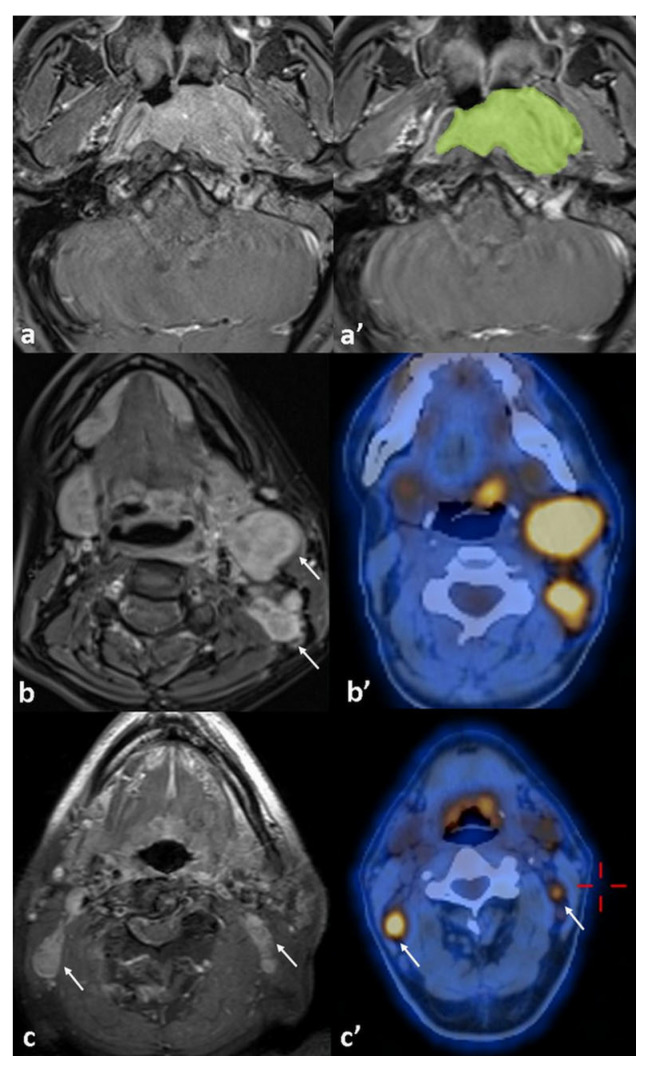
(a) Axial contrast-enhanced T1-weighted MR image shows the primary tumor located on the left lateral wall of the nasopharynx. (a′) Gross tumor volume (GTV) measurement is demonstrated on the same axial slice using the image viewer in syngo.via^®^ VB60 (Siemens Healthineers). The tumor was manually delineated on each axial slice where it was visible, and the software automatically calculated the total volume by summing the segmented areas across slices. The segmented tumor regions were automatically color-coded by the software (green area). (b,b’) Metastatic lymph nodes at left cervical levels IIb and Va are visualized on concurrent MRI and fused ^18^F-FDG PET/CT images (arrows). (c,c’) Bilateral small but pathological lymph nodes are demonstrated on both MRI and fused PET/CT images (arrows).
